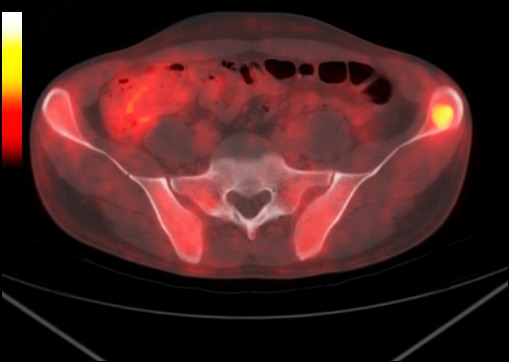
Foyer hyperméabolique (SUVmax bw: 4.1) touchant l'aile iliaque gauche
